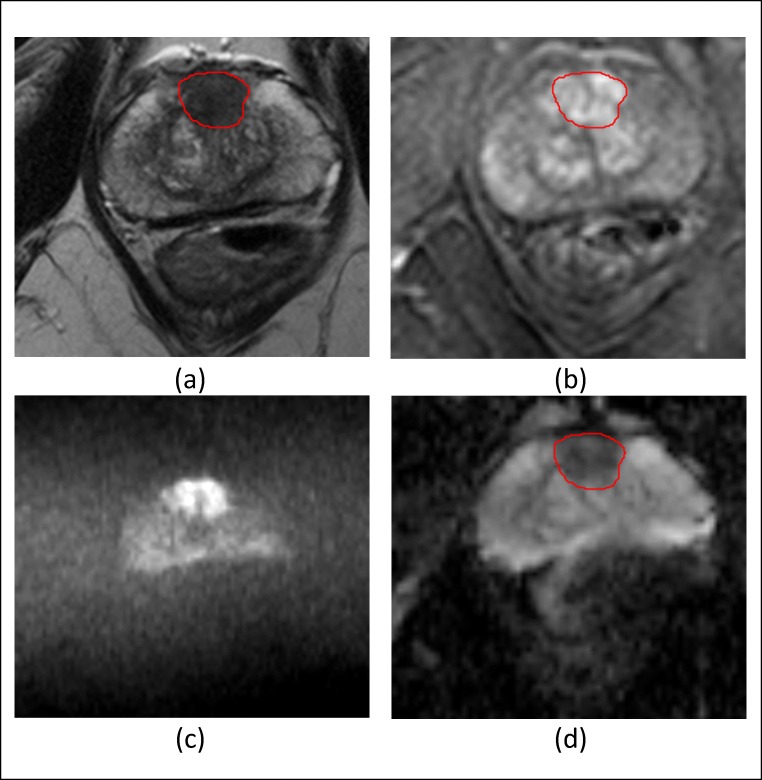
a Axial T2 TSE of a 64-year-old male showing the volumetric contour of a TZ prostate tumor for extraction of mp-MRI parameters. b Axial post gadolinium dynamic contrast-enhanced image. c Axial (*b*) = 2000 mm/s^2^. d ADC “map”
